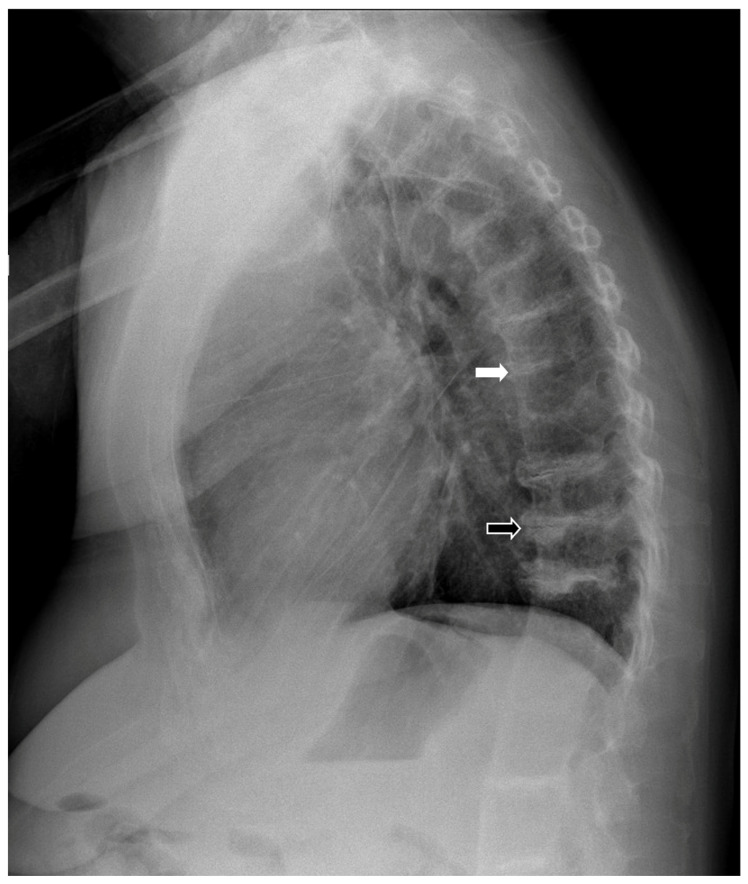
Lateral chest X-ray of the patient taken 2 years ago when she was 81 years old. The spine exhibits degeneration of the intervertebral disc cartilage with end-plate sclerosis (white arrow) and osteophytes typical of lumbar spondylosis (black arrow). The intervertebral bodies may be osteoporotic.
